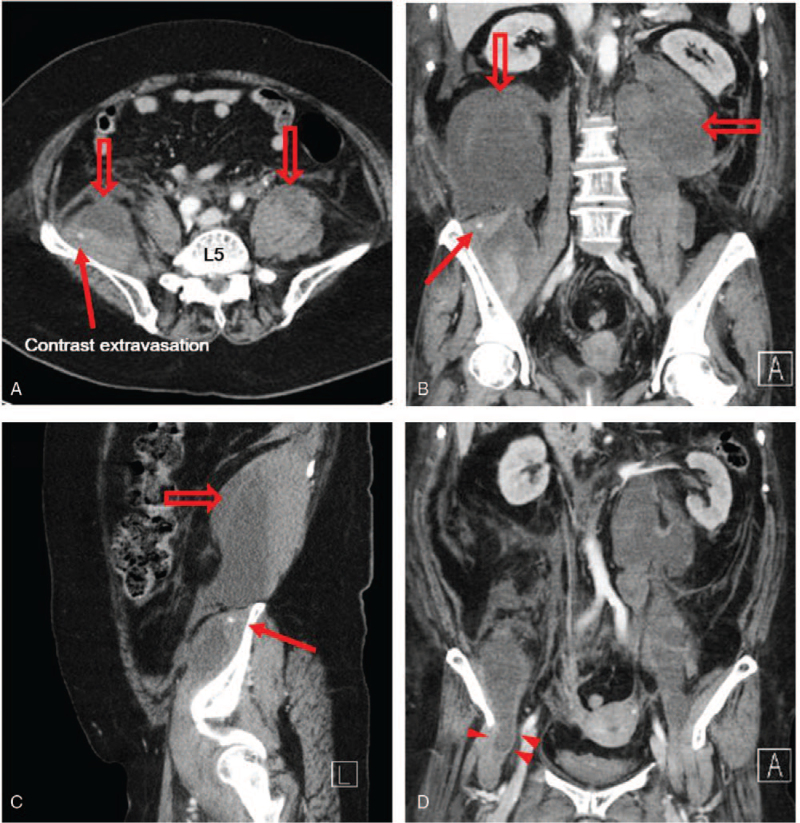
A large number of hematomas in the bilateral retroperitoneal space with active bleeding on abdominal and pelvic CT. (A) Axial image at the lumbar vertebra 5 level; (B) coronal image; (C) sagittal image. The hollow red arrows indicate the large number of bilateral retroperitoneal hematomas involving the psoas and iliacus muscles. The red arrows indicate the contrast extravasation of the right lumbar artery. The red arrowhead indicates the iliacus hematoma extending between the ileum and the inguinal ligament. L5 = 5th lumbar vertebra.
